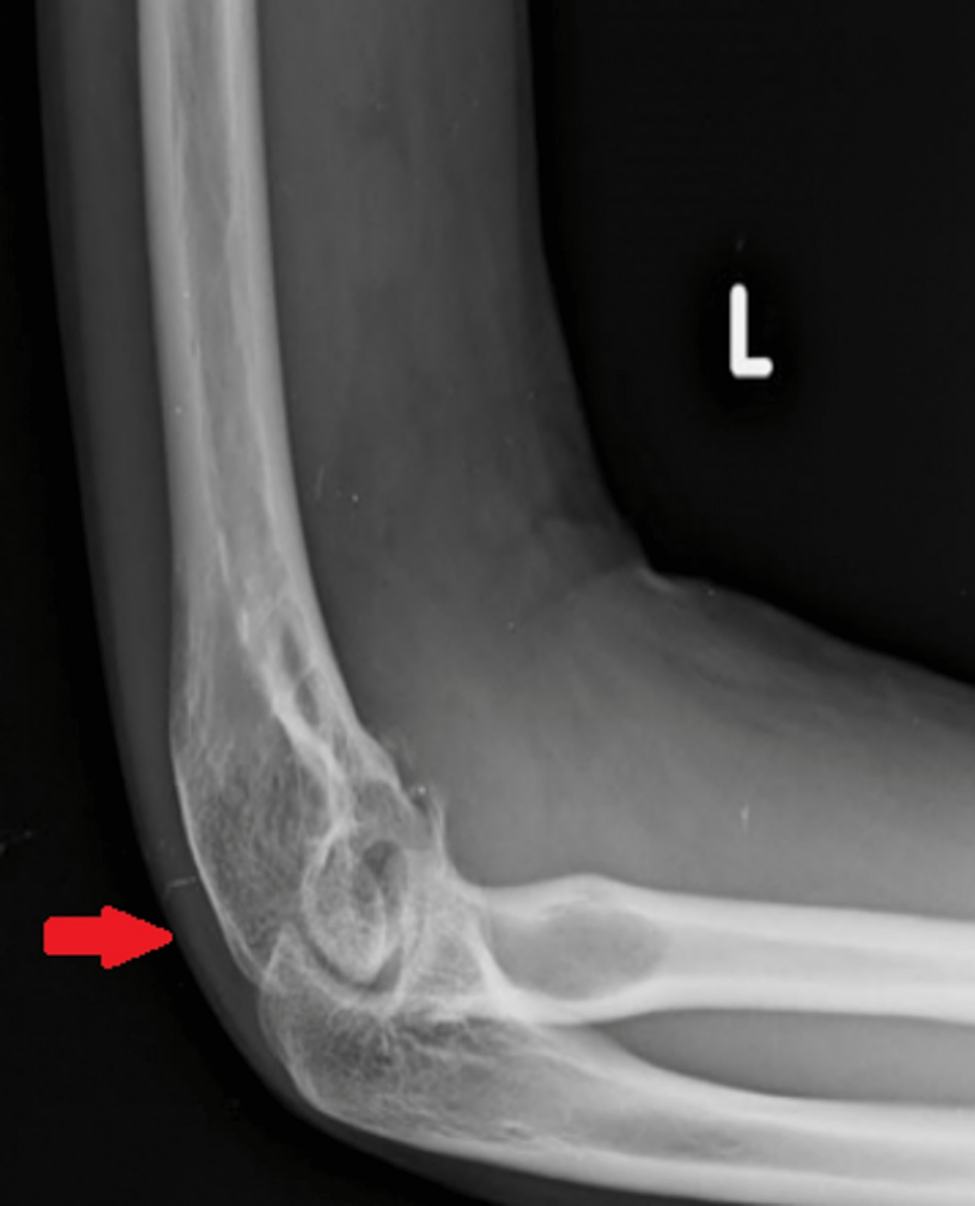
Lateral X-ray of the left elbow joint Arrow showing lytic lesions in the medial epicondyle of the left humerus
